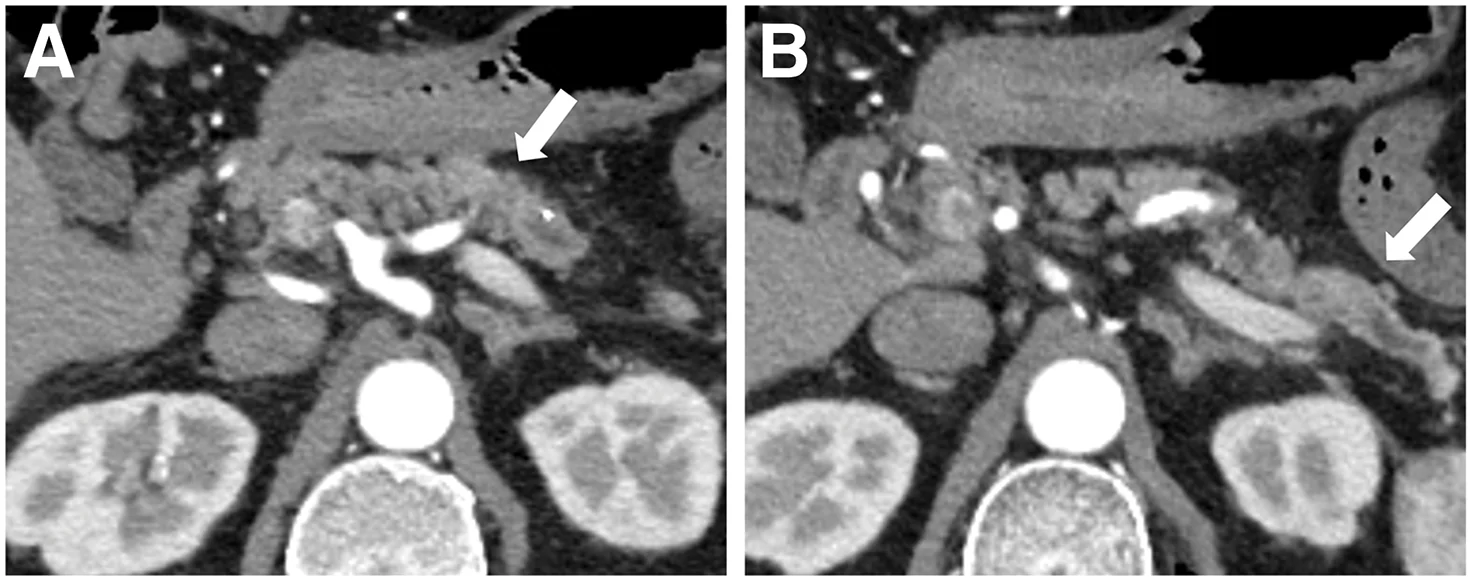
CT imaging findings. (A) A main pancreatic duct stricture in the pancreatic body. (B) A main pancreatic duct stricture in the pancreatic tail with upstream dilatation of the main pancreatic duct.
